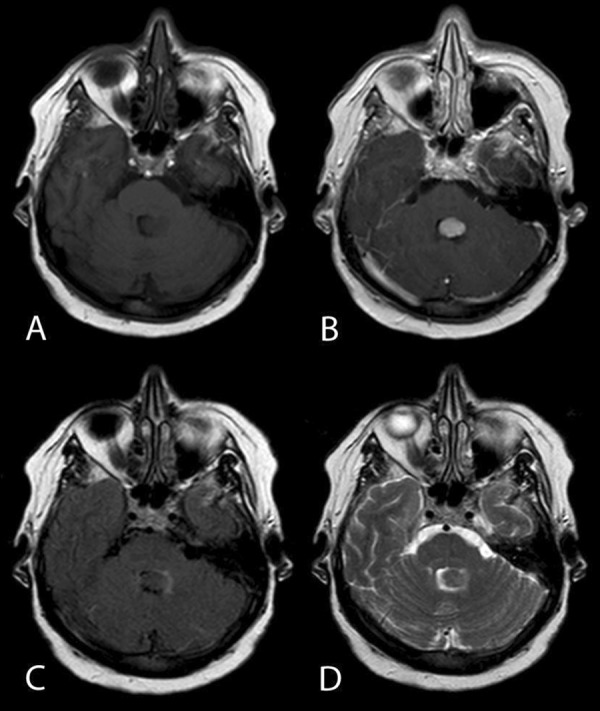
MR images obtained from a 60-year-old woman with diplopia. Axial T1-weighted images without (A) and with (B) contrast enhancement demonstrate a solitary, contrast-enhancing mass lesion within the fourth ventricle. The mass has an isointense signal to cortex on both fluid-attenuated inversion recovery (FLAIR) (C) and T2-weighted (D) pulse sequences.
